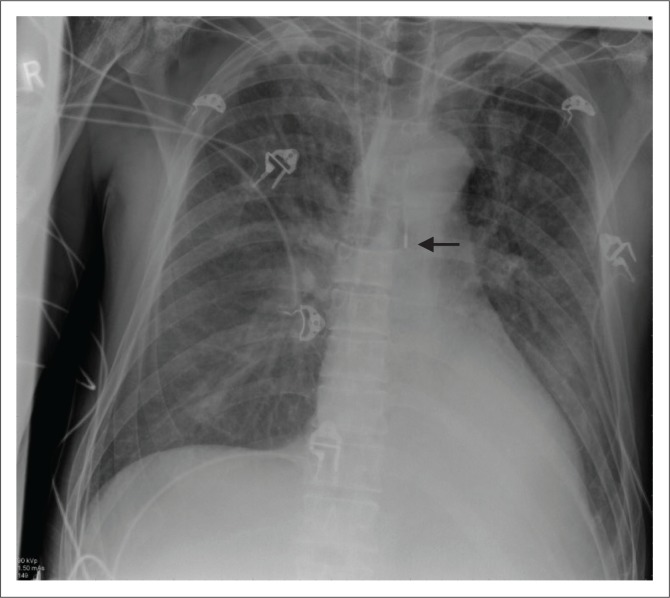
Chest radiograph showing an intra-aortic balloon pump placed in a 53-year-old male with acute post-infarction ventricular septal rupture and cardiogenic shock. Note that the radiopaque tip of the intra-aortic balloon pump (arrow) is at or below the level of the carina and needs to be advanced by 2 cm.
